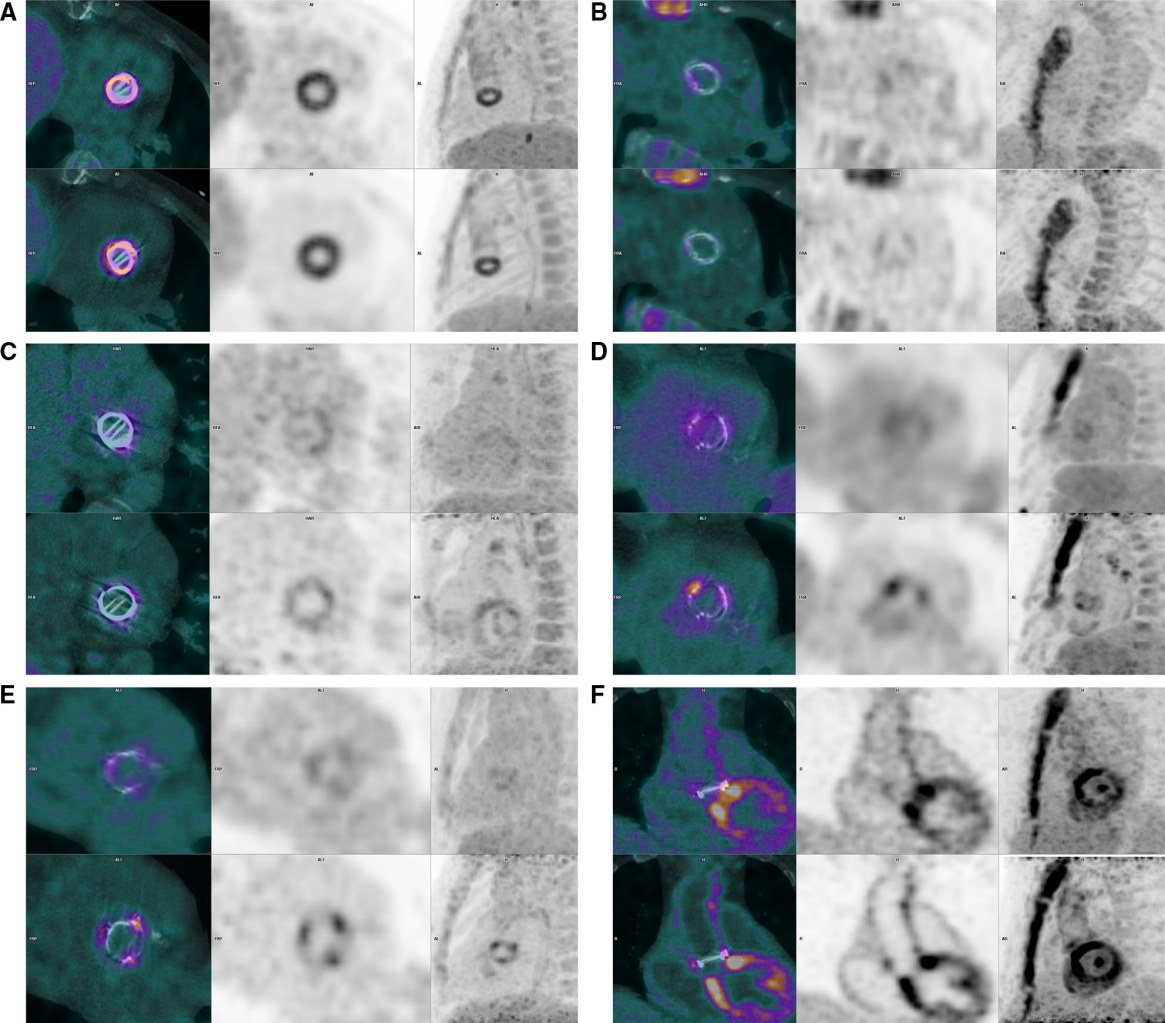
Examples of standard (*top row*) and late (*bottom row*) FDG PET/CT images in six patients. A True positive uptake surrounding a mechanical aortic PHV in both phases. B True negative images with hardly any uptake near the biological aortic PHV in either phase. C True negative standard images with minimal uptake surrounding the mechanical mitral PHV, clearly delineated in the late image. D True positive late phase with focal uptake near the strut of the biological aortic PHV near the right coronary that is not apparent on standard images. Note accumulation of uptake in mediastinal lymph nodes. E False positive late images with exaggerated uptake near all struts of a biological aortic PHV with normal uptake on standard images. F False positive uptake in both phases surrounding the prosthesis after semi-recent Bentall procedure complicated by haematoma. Note the unsuppressed physiological uptake in the myocardium
